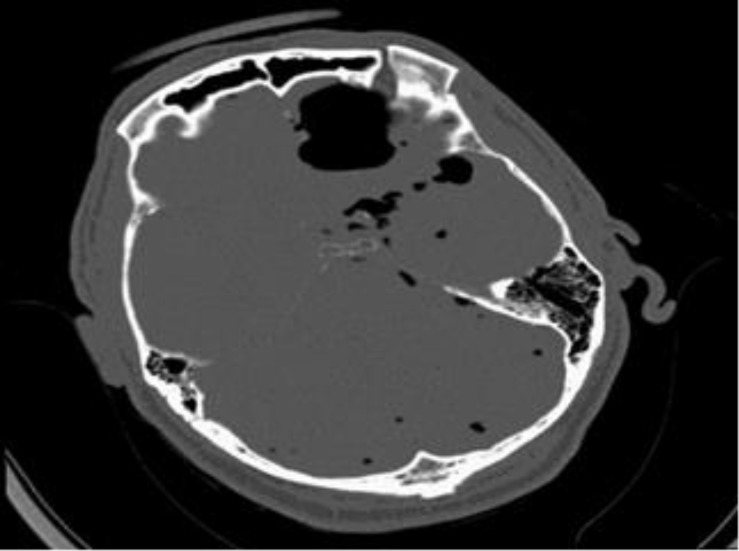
CT of patient on admission demonstrating pneumocranium with old skull fractures
